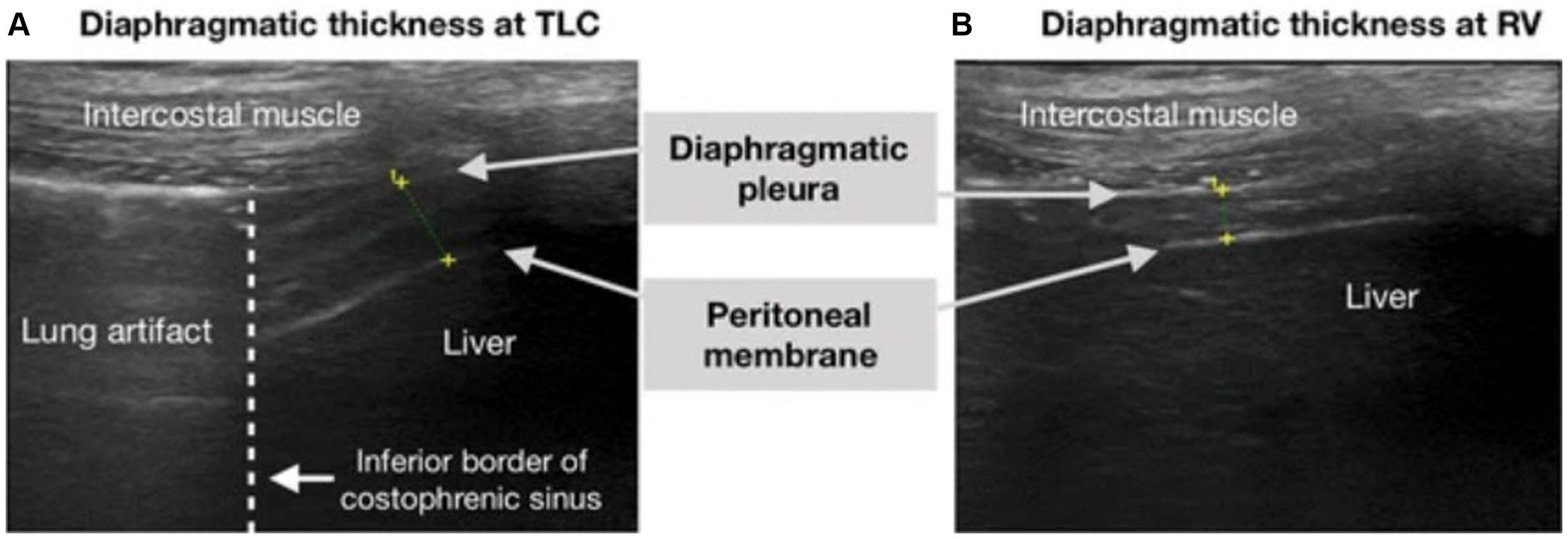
Obtaining diaphragm thickness fraction on ultrasound. Ultrasound image of the diaphragm on B-mode, showing the three layers of the diaphragm (hypoechoic diaphragm bordered by hyperechoic diaphragmatic pleura and the peritoneal membrane). Seen in (A) total lung capacity (TLC) and (B) residual volume, which is equivalent to end-inspiration and end-expiration, respectively. This image was reproduced with the permission of the Creative Commons license. No changes were made to this image. The Creative Commons Public Domain Dedication waiver (http://creativecommons.org/publicdomain/zero/1.0/) applies to this image (41).
